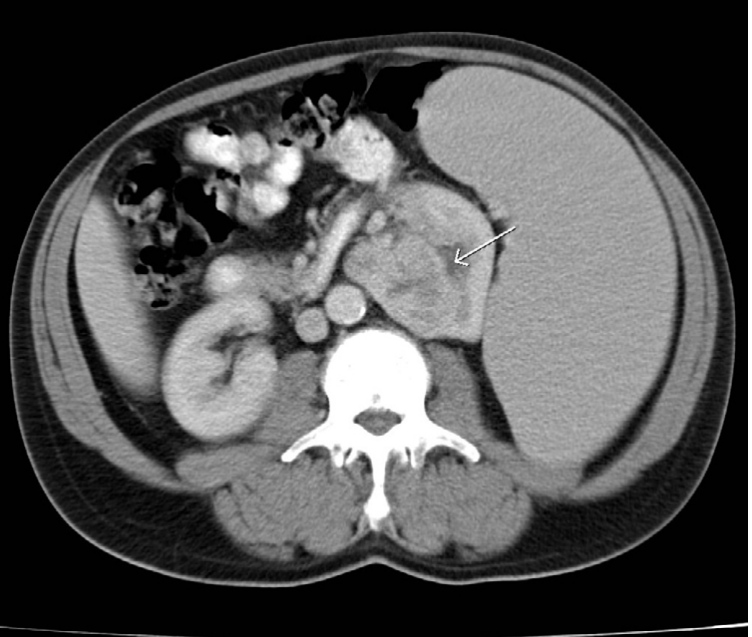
CT showing a left renal carcinoma (white arrow) and splenomegaly.
